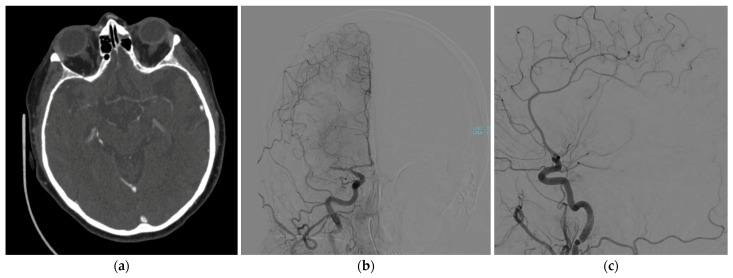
Brain CTA showing a complete occlusion of the right middle cerebral artery in (a) axial view; confirmed during a cerebral angiography: (b) coronal and (c) sagittal views.
